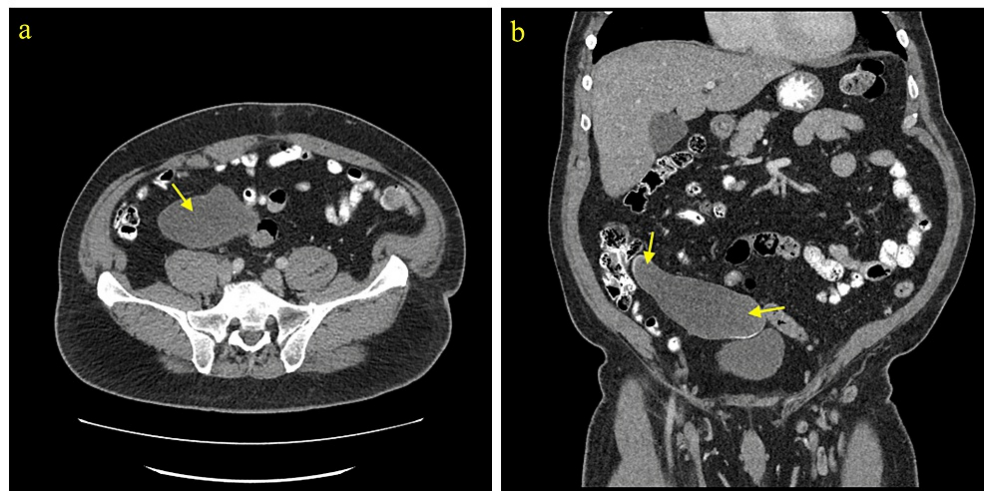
CT abdomen and pelvis with contrast (Case 3). a) Axial view showing a cystic lesion in the right abdomen. b) Coronal view showing a RLQ tubular cystic mass with heterogeneous mural calcifications. Pathology of the mass showed LAMN. RLQ, right lower quadrant; LAMN, low-grade appendiceal mucinous neoplasm
